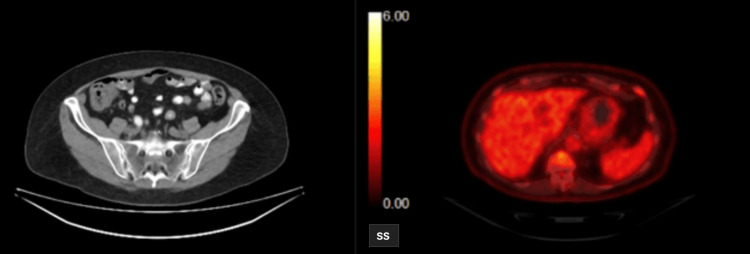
After six cycles of chemotherapy, CT and PET scans demonstrated no FDG-18 avid malignancy, including no presence of cecal mass. FDG-18: fluorodeoxyglucose-18; PET: positron emission tomography.
